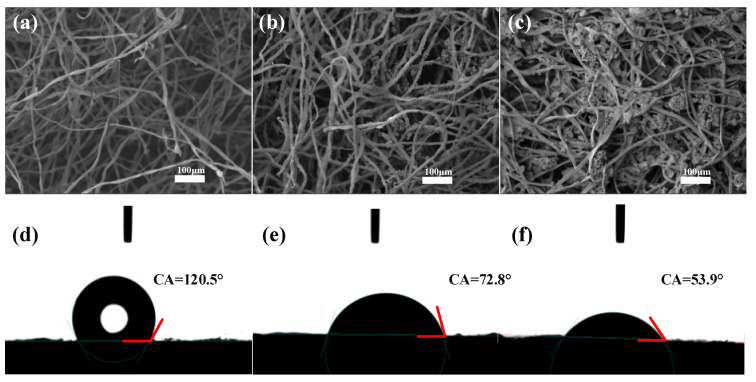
SEM images of the (a) CT, (b) CT-Fe and (c) CT-Fe-PVA-DM; contact angles of (d) CT-Fe, (e) CT-Fe-PVA and (f) CT-Fe-PVA-DM.
